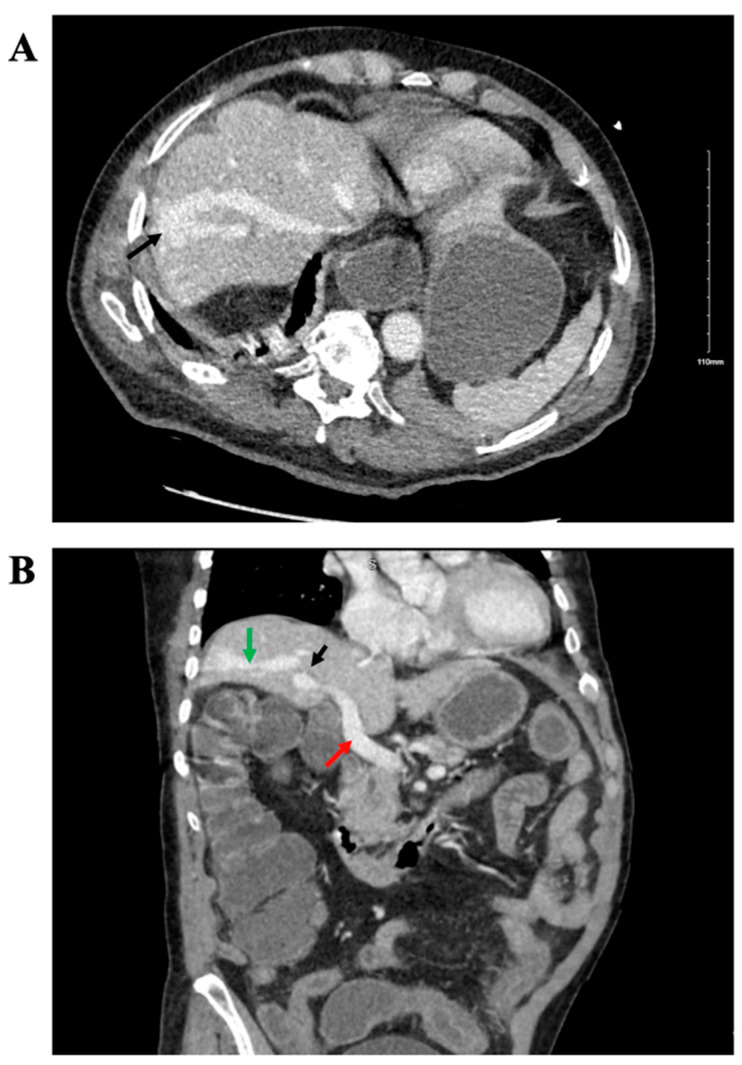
Computed tomography (CT) imaging with contrast demonstrated a portosystemic shunt. (A) Axial view of CT abdomen/pelvis highlighting a stellar dilated vascular lesion at the right hepatic lobe (black arrow). (B) Sagittal view of CT abdomen/pelvis showing communication between portal vein (red arrow) and hepatic vein (green arrow). The location of the shunt is indicated by the black arrow.
